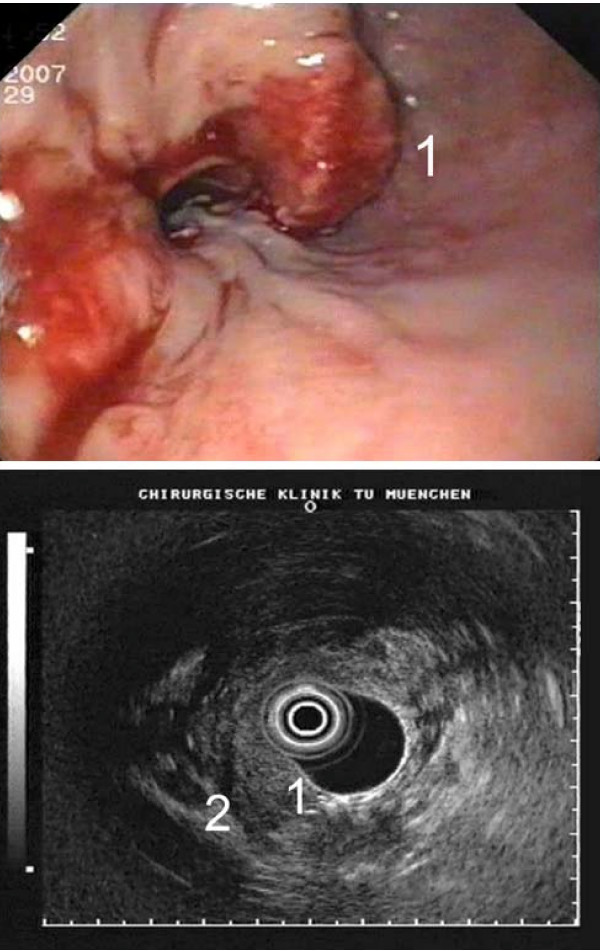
Endoscopy and endosonography of the oesophageal tumor with infiltration of all mucosal layers (1) and peritumoral lymph nodes (2).
